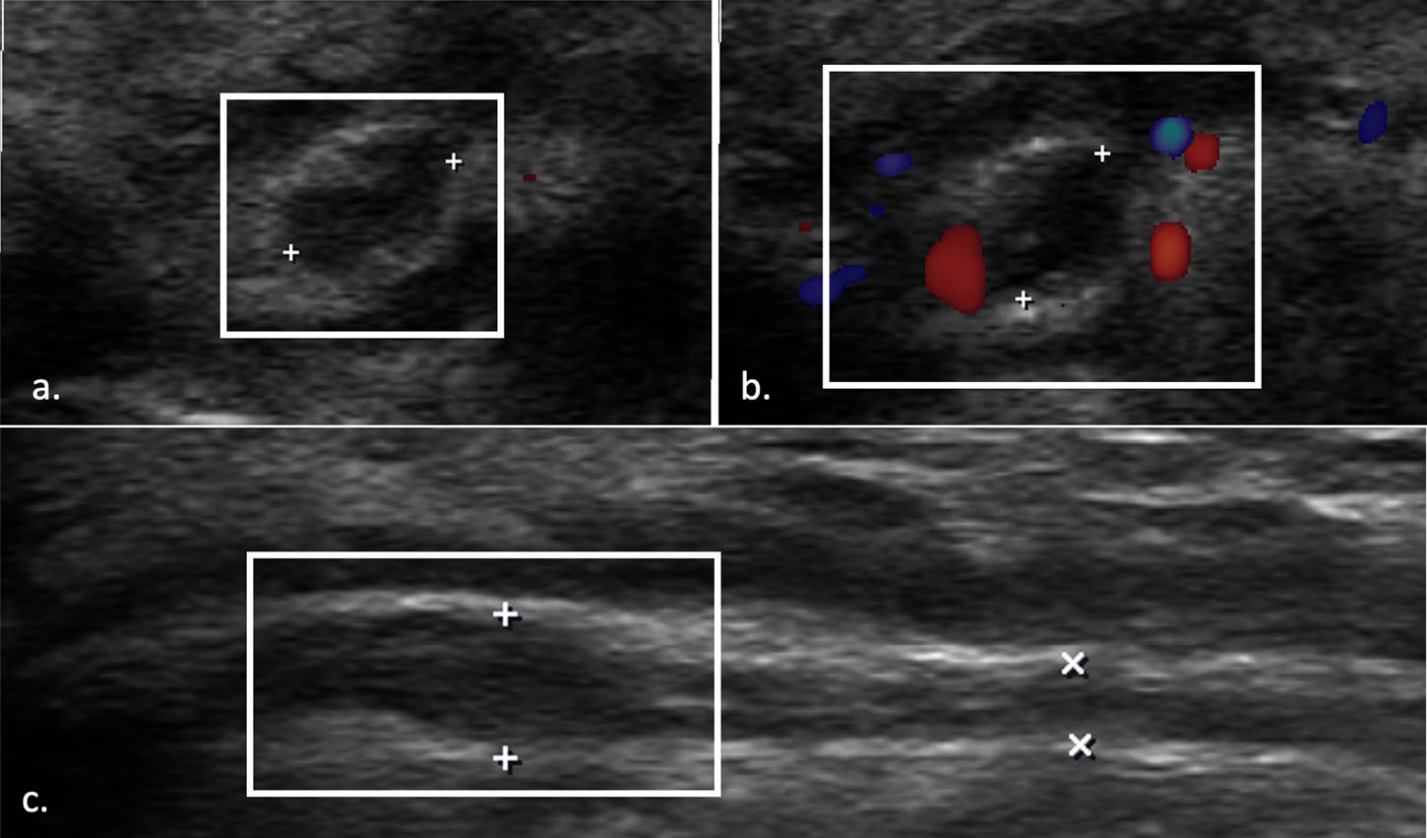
US of cubital tunnel syndrome. Transverse axis (a), color Doppler (b), and longitudinal axis (c) US images of UN within the cubital tunnel. The nerve is focally thickened with loss of fascicular architecture and hypoechoic appearance. There is increased perineurium vascularity on color Doppler. *Case courtesy of Dr. Luca De Flaviis, Milano, Italy*
